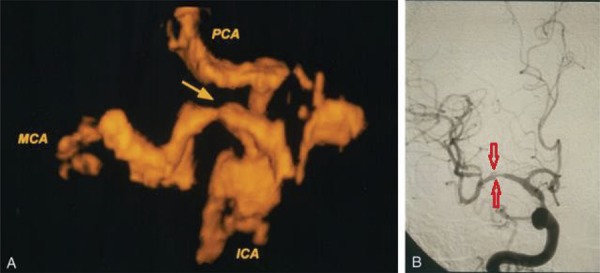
Anteroposterior images of an intracranial stenosis. A. A 3D power doppler image shows the circle of Willis with severe stenosis (arrow) in the MCA. ICA=internal carotid artery; MCA=middle cerebral artery; PCA=posterior cerebral artery. B. An angiogram confirmed severe stenosis (arrows) in the MCA stem.
